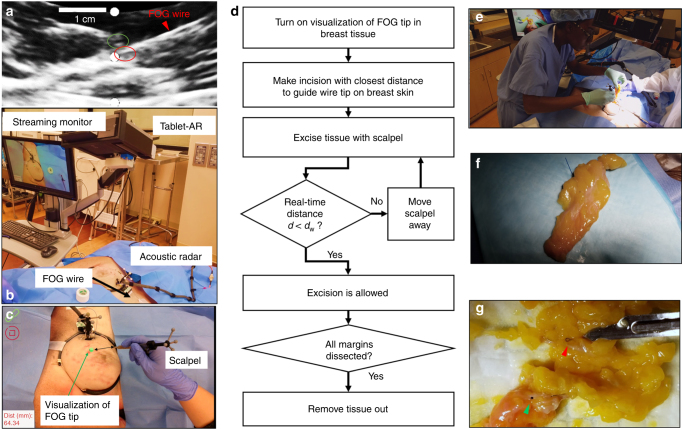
Excision of a “pseudo tumor” marker by a biopsy clip in the breast of a female human cadaver using the fiber optoacoustic guide and the tablet-AR system. a Ultrasound image that confirms the implantation of the guide wire and biopsy clip in the tissue. The FOG tip (red circle) is close to the implanted biopsy clip (green circle). b Setup of the system in the excision experiment. c Visualization of the FOG tip in the breast tissue (green dot) and the real-time distance of the scalpel tip to the FOG tip on the AR display. d Protocol of the excision of the “pseudo tumor” in the experiments. e Photo of the board-certified breast cancer surgeon using the system to excise the tumor. f Photo of the excised “pseudo tumor”. g Photo of the cut-open excised tissue, in which the implanted biopsy clip and the FOG tip are inside, which represents a successful excision of the “pseudo tumor”. The green arrow shows the FOG tip, and the red arrow shows the biopsy clip
